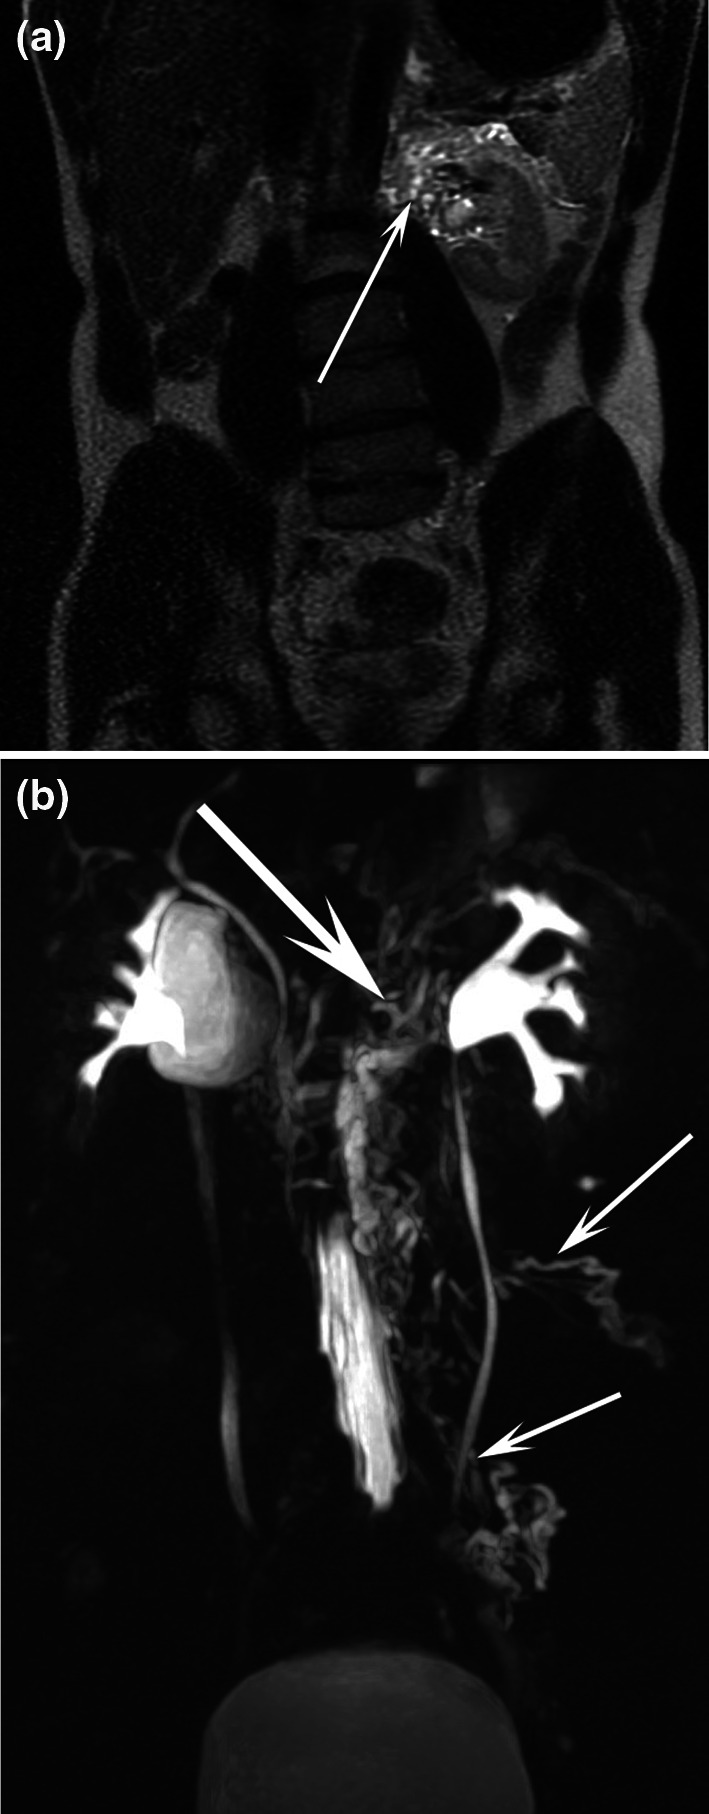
37-year-old male patient with chyluria. Coronal T2-weighted MR image a demonstrated abnormal lymphatic vessels (arrow) in touch with the left renal pelvis, which was better seen by (b) coronal MR lymphography with MIP reconstruction. Dilated lymphatic vessels close to the left ureter (short arrows) were also demonstrated
